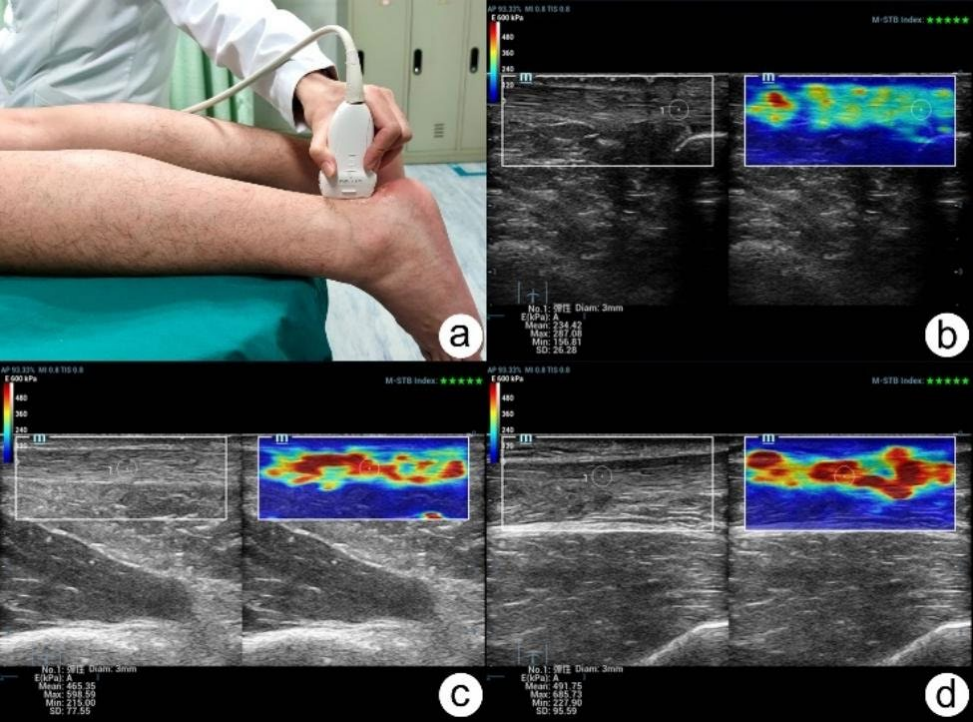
AT ultrasonography. (a) Subject position during the examination. Each subject was requested to lie on the examination bed in prone position, with both feet suspended over the edge of the bed fully relaxed. (b) STE examination of the distal segment of AT. The measured Emean value of the distal segment of AT was 234.42 kPa. (c) STE examination of the middle segment of AT. The measured Emean value of the middle segment of AT was 465.35 kPa. (d) STE examination of the proximal segment of AT. The measured Emean value of the proximal segment of AT was 491.75 kPa. AT, Achilles tendon; STE: Sound touch elastography; Emean: mean Young ‘s modulus
